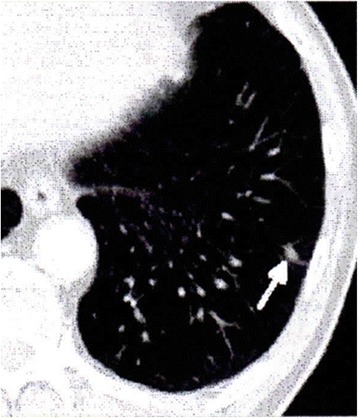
Computed tomography of case 2. An image before formalin-fixed tumor vaccine therapy shows a mass in the left lung (arrow)
